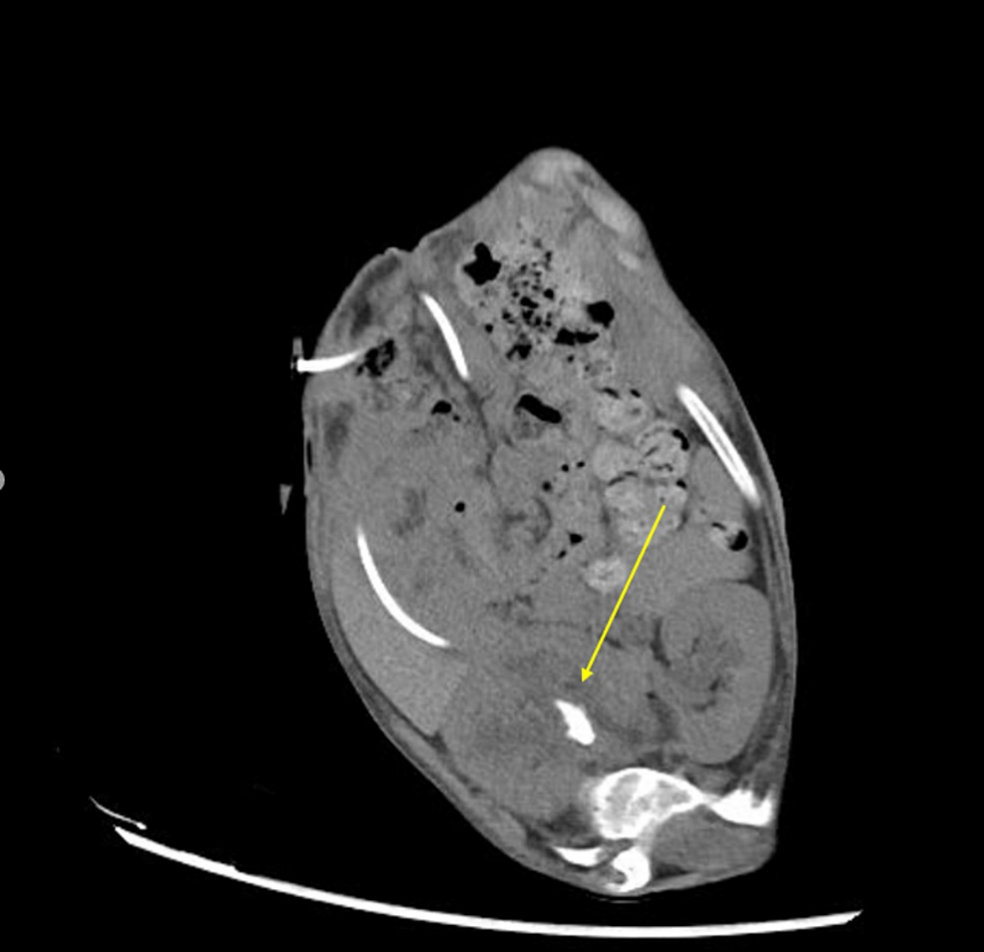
An axial view of the CT scan showing a radiopaque staghorn stone (yellow arrow) in a malrotated kidney with mild hydronephrosis. CT: computed tomography.
